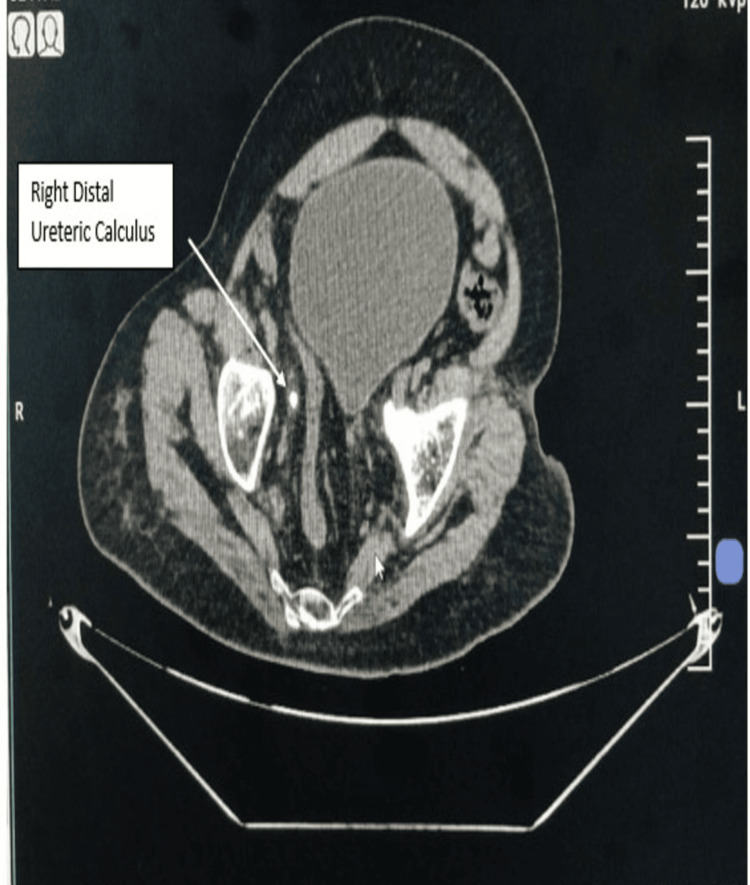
CT KUB axial section demonstrating distorted pelvic anatomy. Non-contrast CT of the kidneys, ureters, and bladder, axial section, demonstrating distorted pelvic anatomy with altered spatial relationships between the urinary bladder and surrounding structures. The white arrow indicates the right distal ureteric calculus. These findings contributed to difficulty in ureteroscopic navigation and access to the distal ureter. CT KUB: Computed Tomography of the Kidneys, Ureters, and Bladder.
